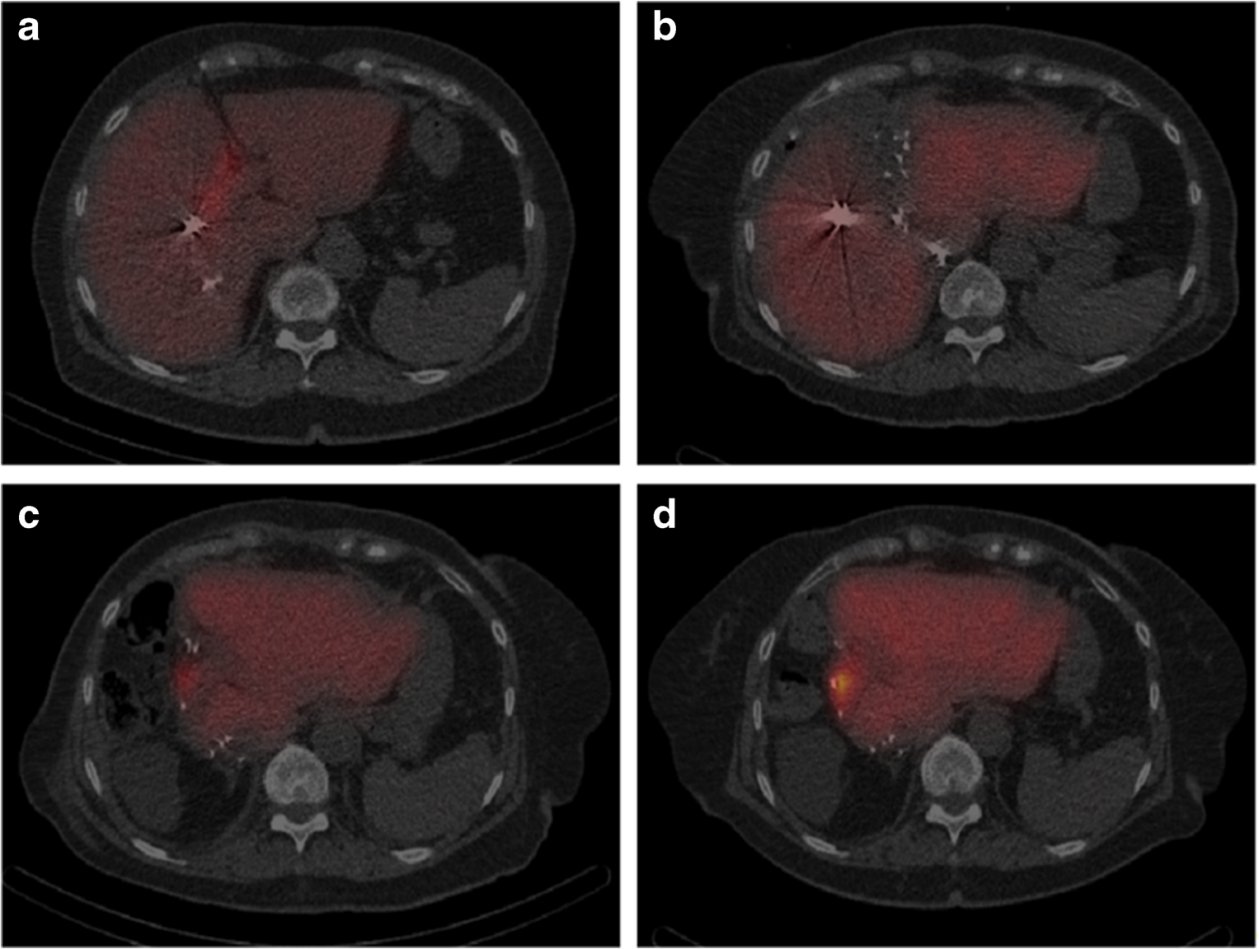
HBS with SPECT/CT in a patient with insufficient growth after previous PVE before stage 1 (a), on day 6 after stage 1 (b), day 7 (c), and day 28 (d) after stage 2
